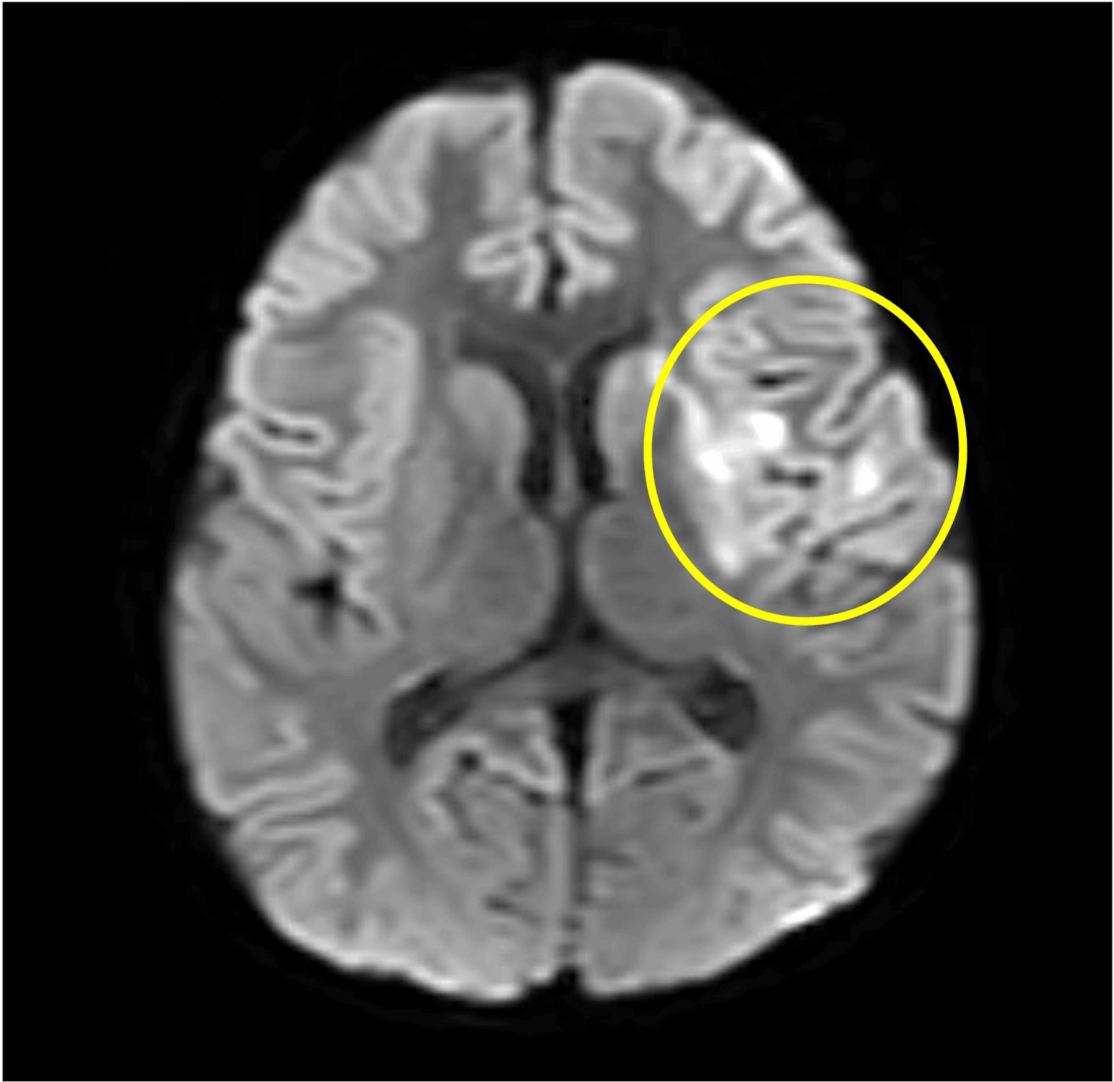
Diffusion-weighted magnetic resonance imaging (3.0T, b=1000 s/mm², slice thickness 5 mm) performed 24 hours after onset of cerebral infarction. The image shows high-signal-intensity lesions in the middle cerebral artery territory (circled area).
